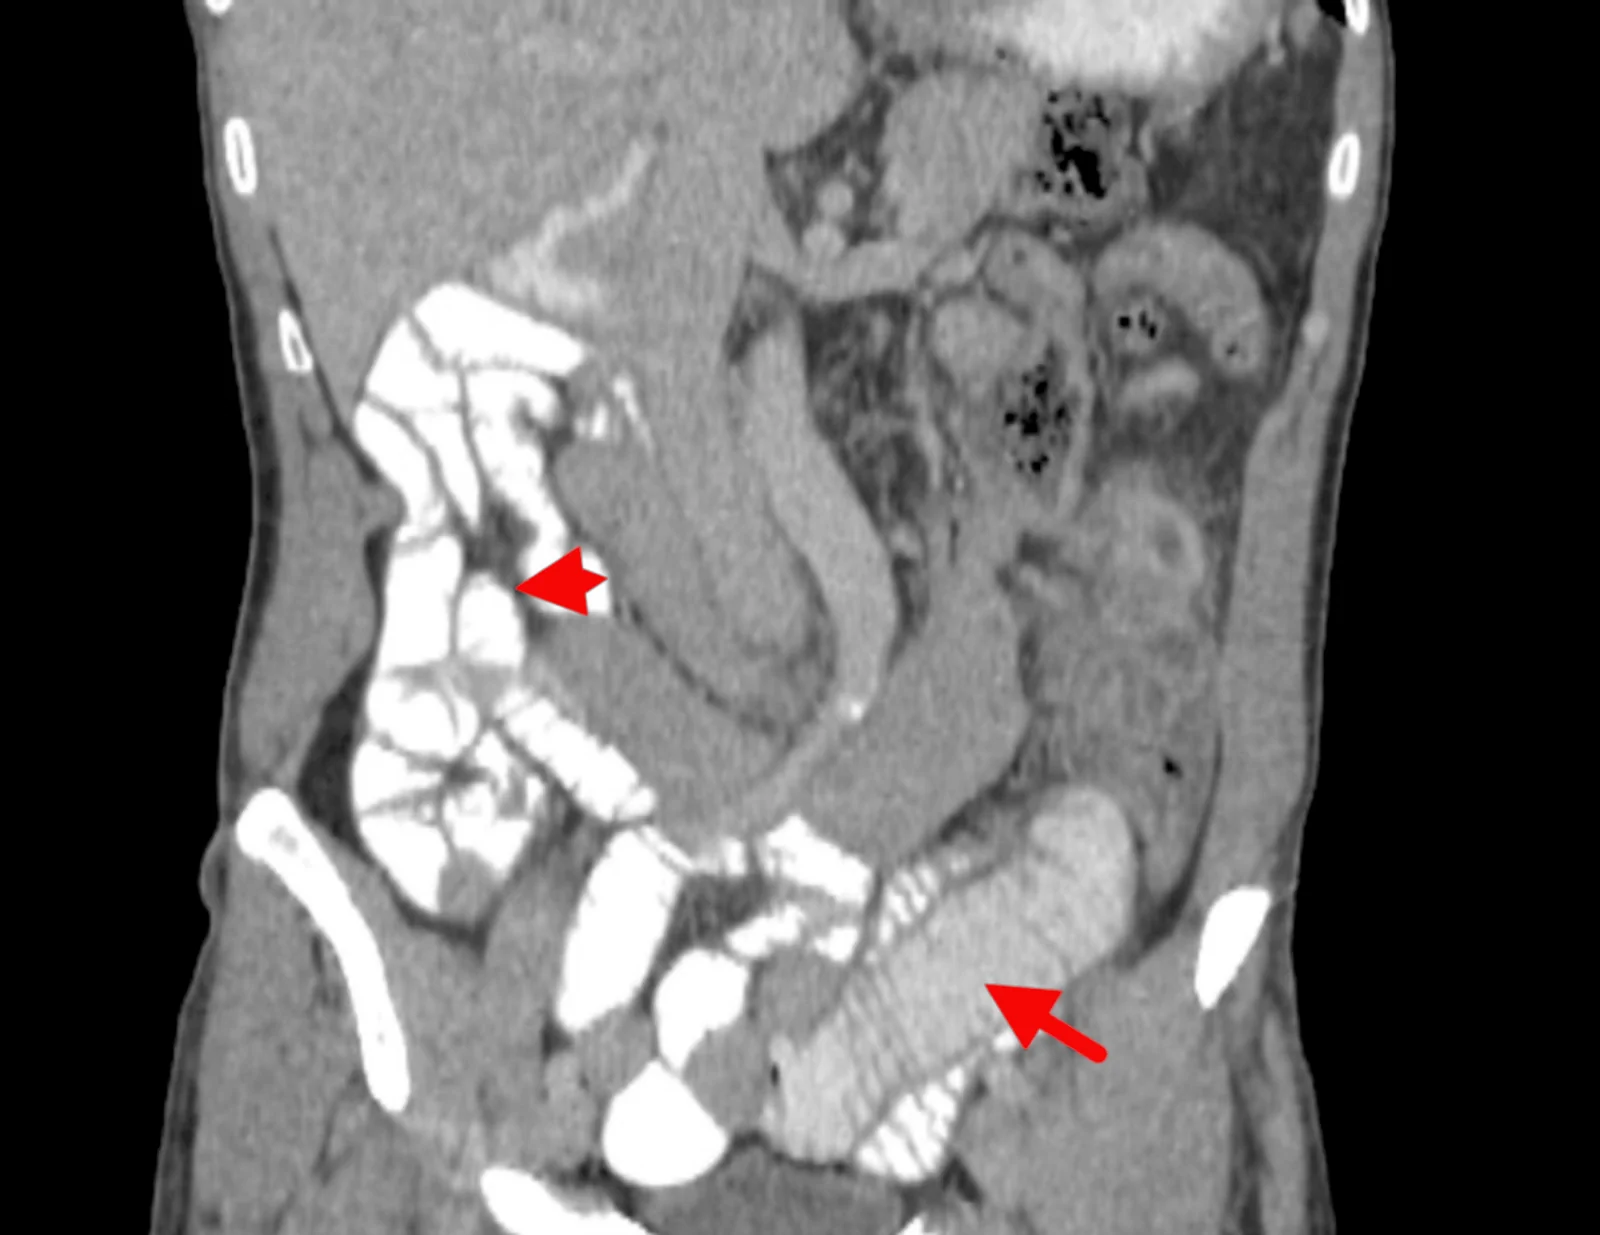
Intestinal nonrotation on coronal CT Coronal contrast-enhanced CT image demonstrating an abnormal bowel arrangement, with the small bowel loops (arrowhead) predominantly located in the right abdomen and the large bowel loops (arrow) predominantly located in the left abdomen, consistent with intestinal nonrotation.
